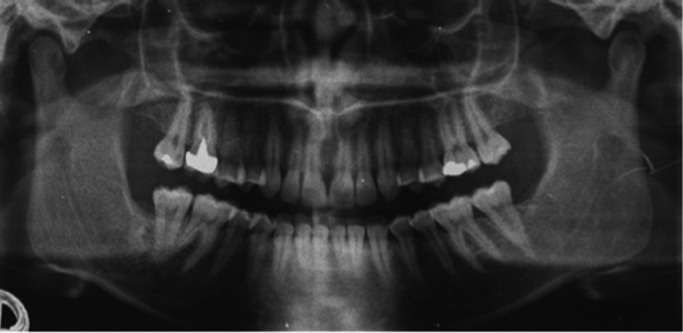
Five years orthopantomographic follow-up. The second molar seems slightly aligned.
